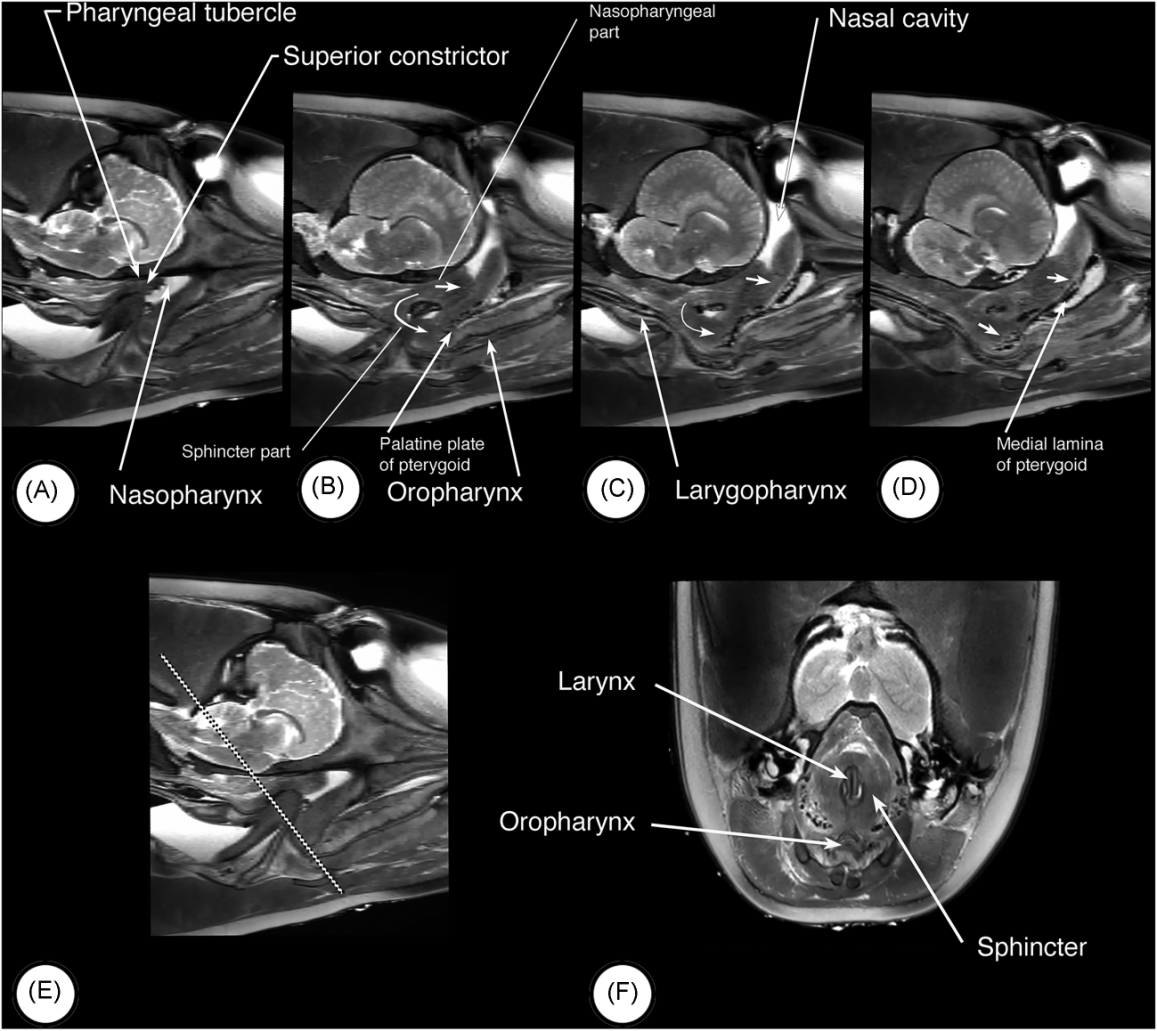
MRI scans showing the position and parts of the superior constrictor of the pharynx in a pacific white sided dolphin. The scans begin in the mid-sagittal plane (A) and progress laterally in (B), (C), and (D). The pharyngeal tubercle in (A) marks the approximate site of origin of the superior constrictor. As the muscle is followed in the sequential scans shown in (B) through (D), it has two parts: a nasopharyngeal part that attaches to the vertical aspect of the medial lamina of the pterygoid and is a component of the nasopharyngeal wall, and a sphincter part that is more ventral, forms a major component of the palatopharyngeal sphincter, merges with the soft palate and attaches to the horizontal part of the pterygoid process. The hatched line in the mid-sagittal section shown in (E) is the approximate position of the oblique section shown in (F). In (F), the sphincter surrounding the rostral end of the larynx just ventral to laryngeal inlet is evident.
